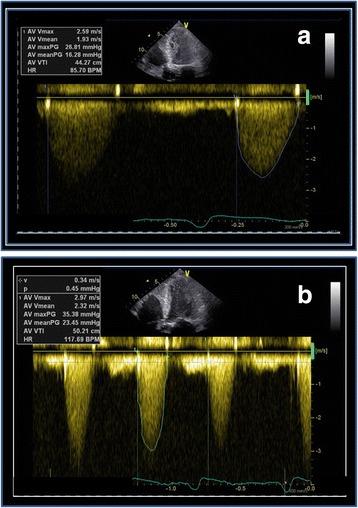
a Stress echocardiography at rest conditions. AV mean pressure is calculated as 16 mmHg. b Under stress conditions (20 gamma dobutamine), AV mean pressure did not exceed 23 mmHg
